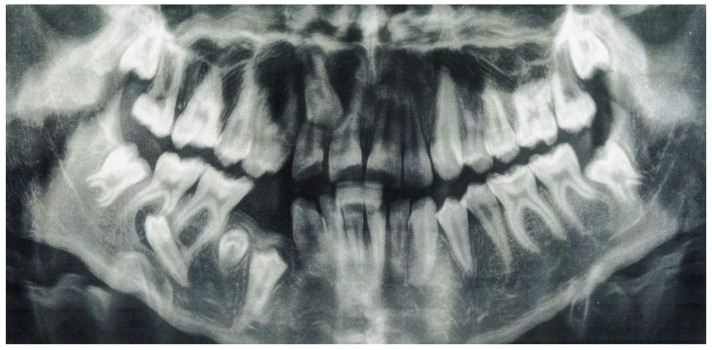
Panoramic radiograph, taken one year later, when patient was 13 years old, showing the germ of the third additional tooth located in the standard position of the 45 tooth—between the crown of the impacted tooth 44 and the mesial root of the tooth 46. The further migration of the tooth 45 is visible—the crown can be seen in the projection of the apex of the mesial root of the tooth 47. Furthermore the impacted tooth 13, located between 1/3 cervical part of the roots of the teeth 12 and 11, is also visible.
